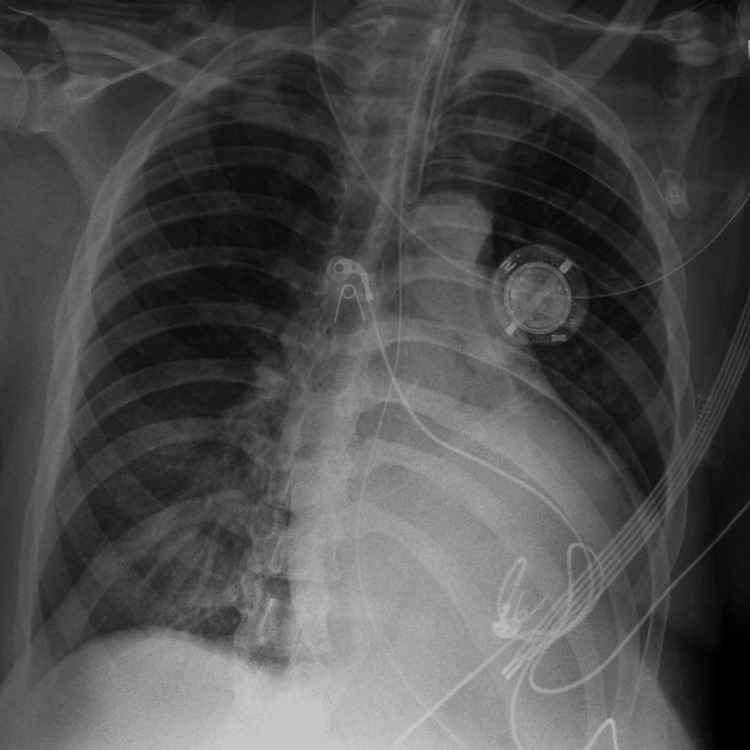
Reexpansion after bronchoscopic airway clearance Chest radiograph showing reexpansion of the left lung and correction of mediastinal shift following removal of extensive mucus plugging
